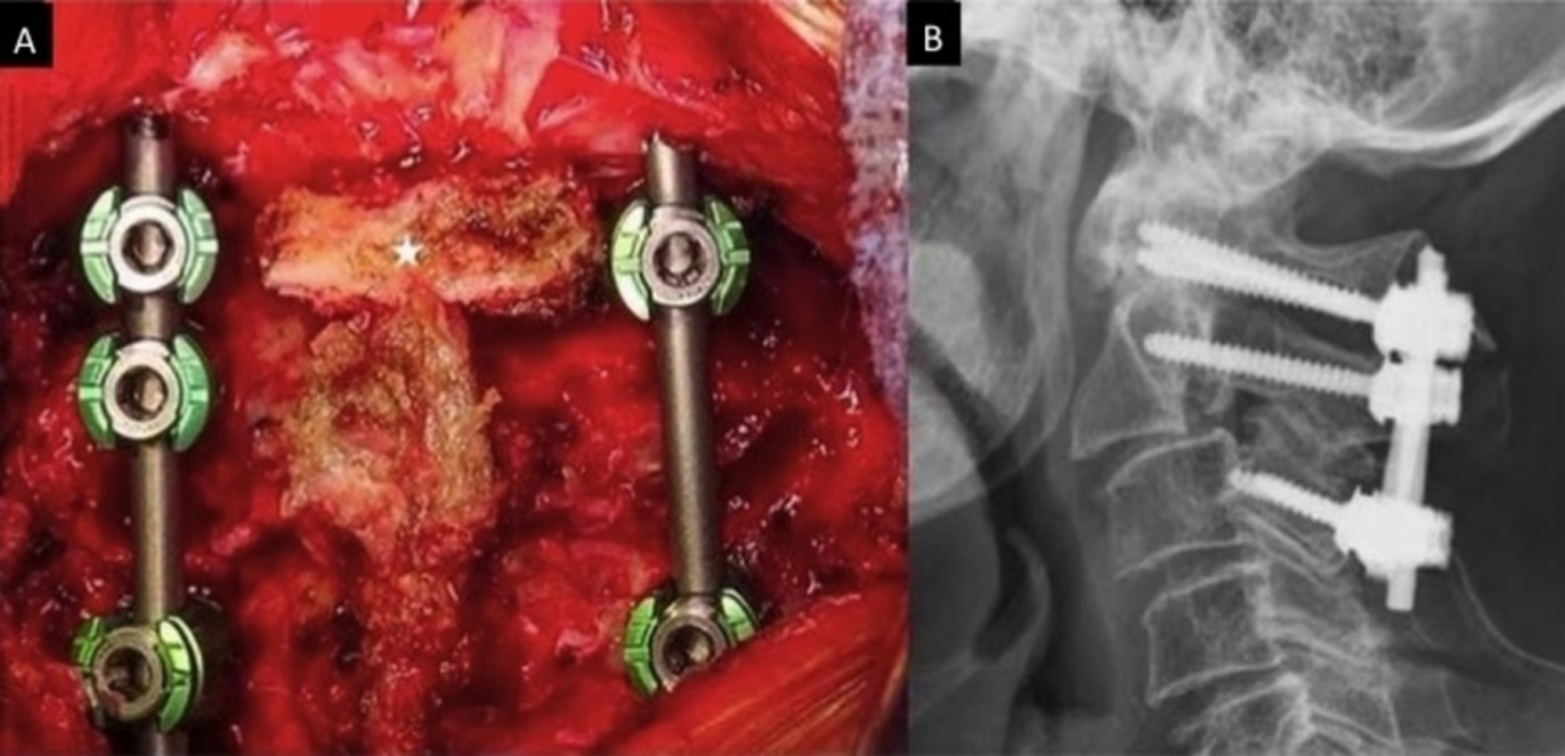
Final construct and postoperative X-ray. (A) Intraoperative photograph of the final C1-3 construct. A small piece of iliac crest graft (star) was placed in between the decorticated C2 and C1 laminae for fusion material. (B) Postoperative lateral X-ray showing stability of the construct post-mobilization.
